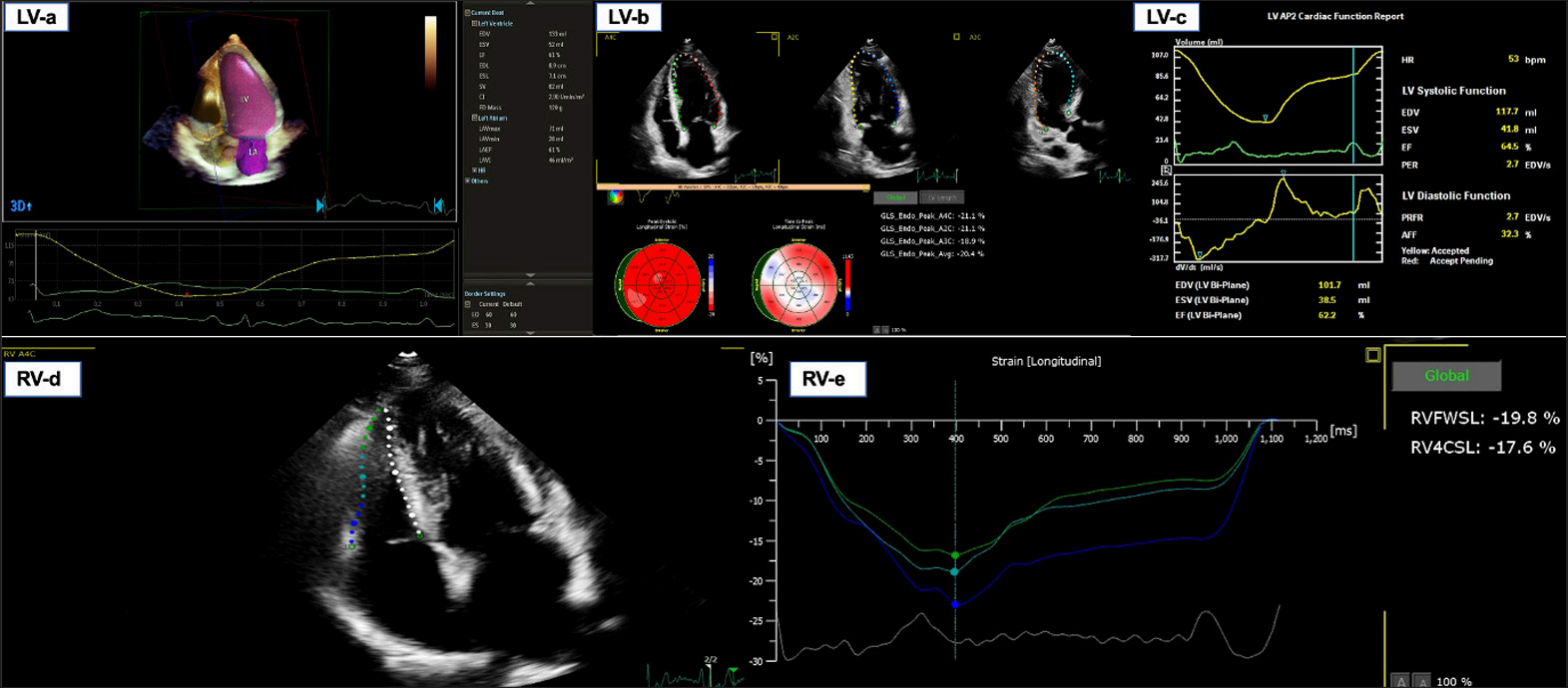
Left ventricle and right ventricle echocardiographic systolic analysis.
